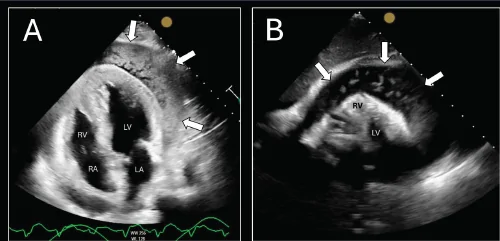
Cardiology ultrasound showing an apical four-chamber view (A) and a subxiphoid view (B) of the patient’s heart with visualization of the left atrium (LA), left ventricle (LV), right atrium (RA), and right ventricle (RV). There is a large mixed-echogenicity pericardial effusion (*white arrows*).
